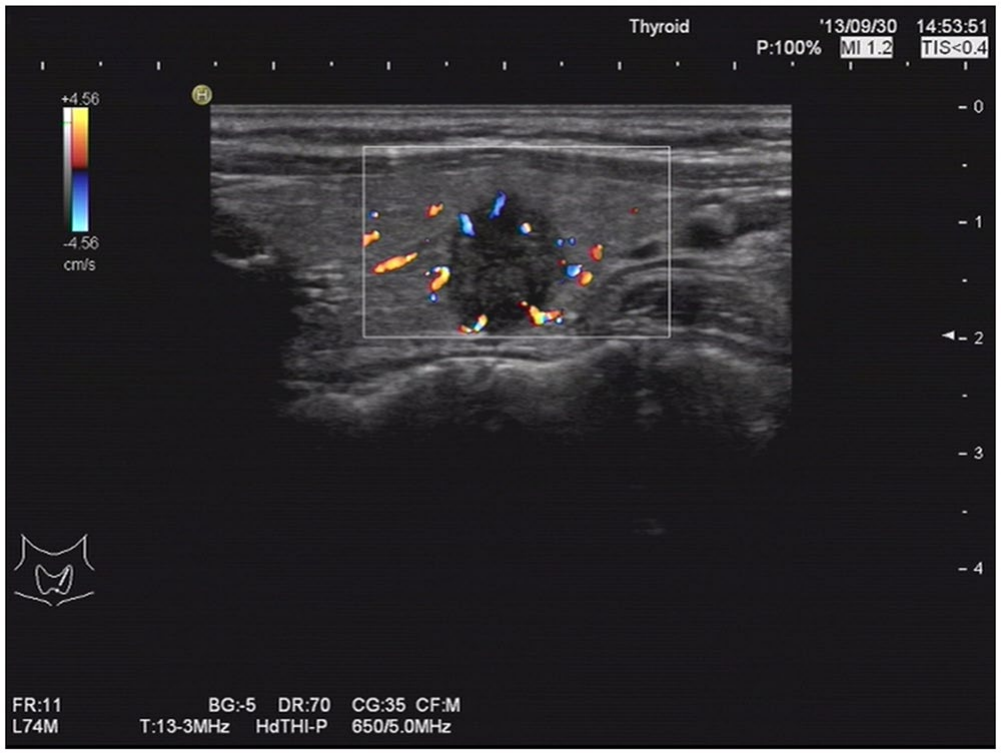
The left thyroid nodular showed increased nodular blood flow signals and blood supply rich and penetration from capsule by Doppler ultrasonography in the same patient.
